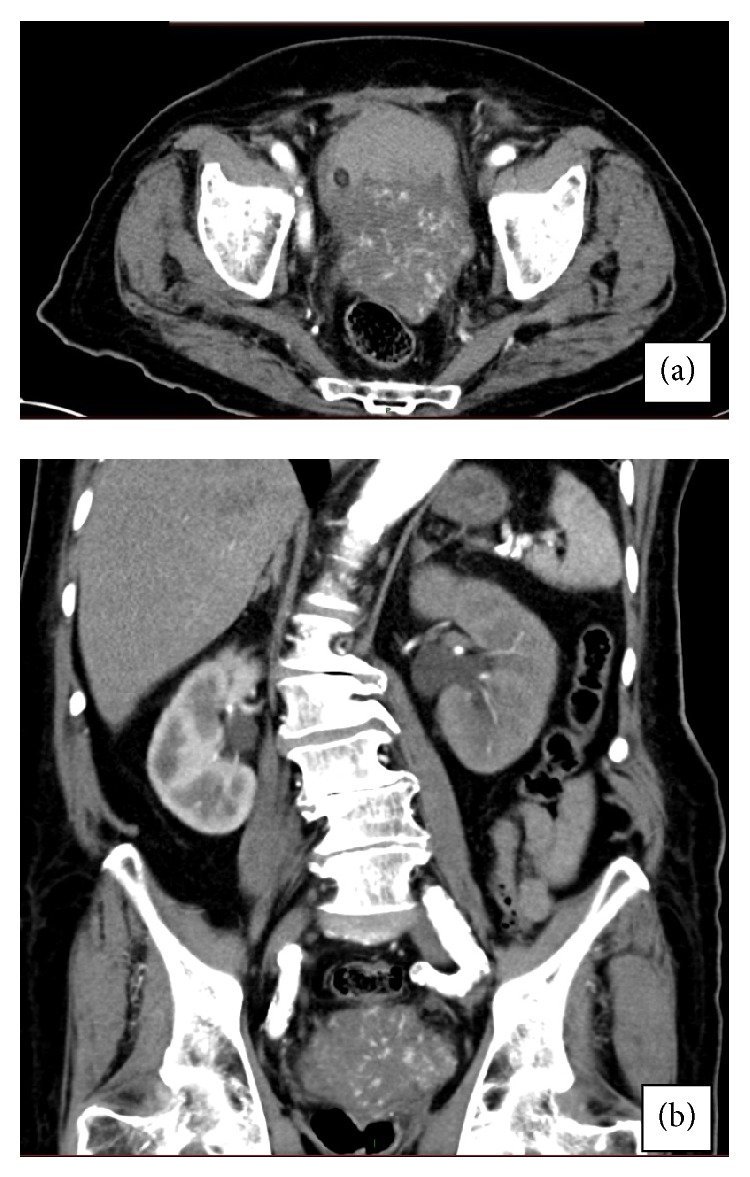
Computed tomography of our patient. (a) Highly vascular mass originating from the left bladder wall, but a prostatic origin could not be absolutely rolled out by CT. (b) Hydronephrosis on the left side and coronal view of the mass.
